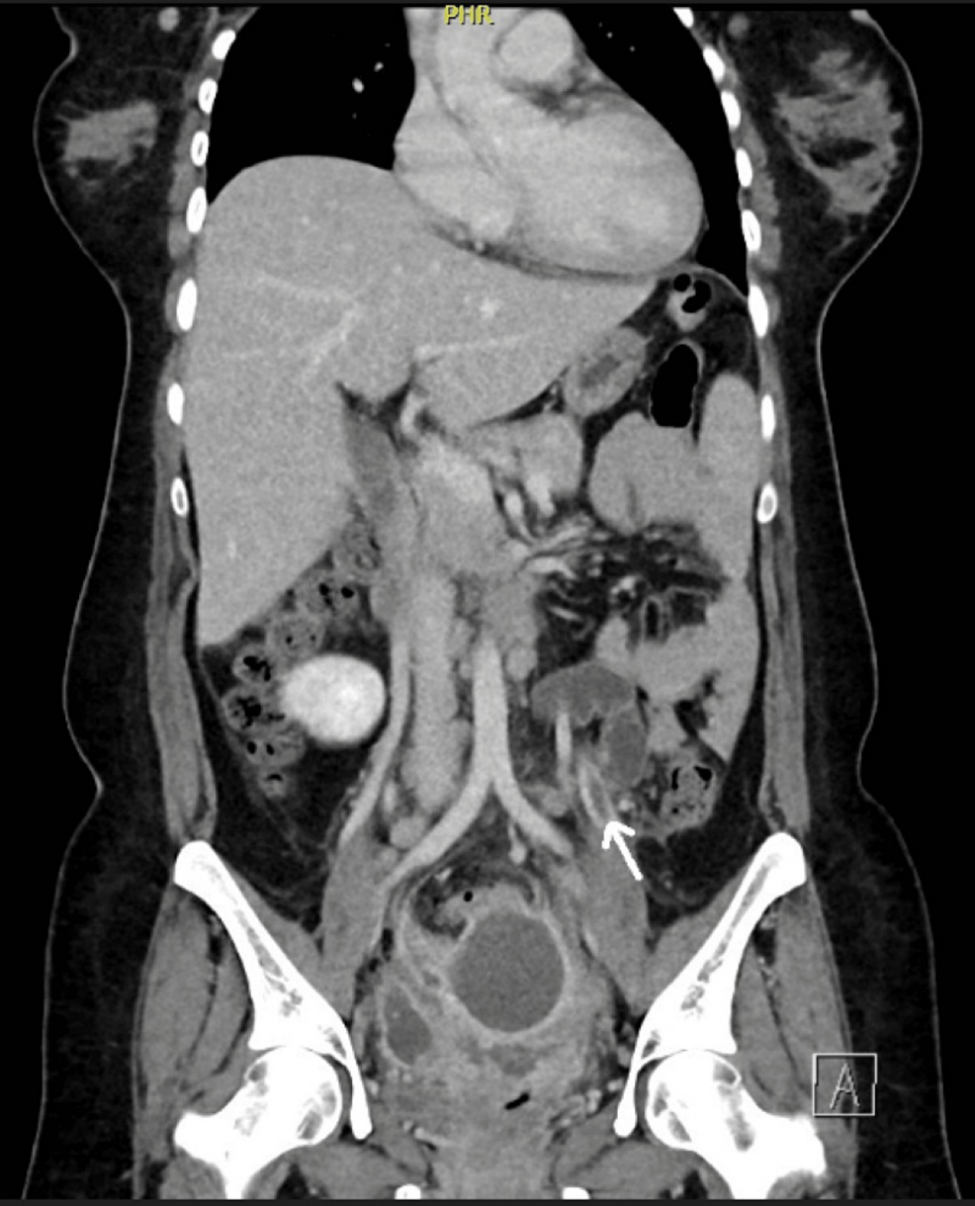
CT scan of the pelvis in Case 2, showing left ovarian vein thrombosis (arrow)
